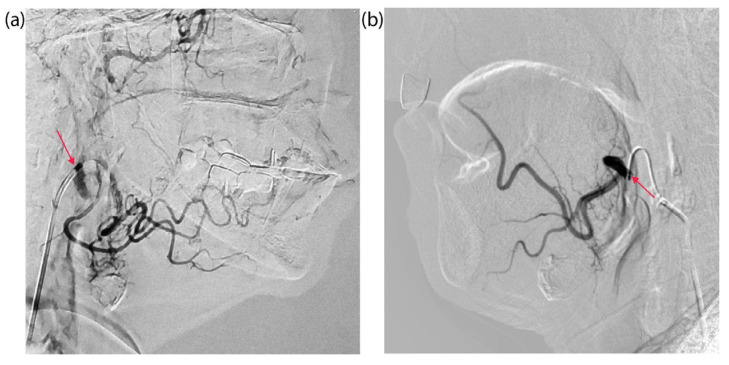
Catheterization of the arteries supplying the tumor under CT guidance: (a) patient KIA—installation of a microcatheter in the projection of the mouths of the facial and lingual arteries extending from the right ECA; (b) patient PCM—selective microcatheterization of the left facial artery through the left ECA using a microcatheter. The arrow indicates the location of the microcatheter.
